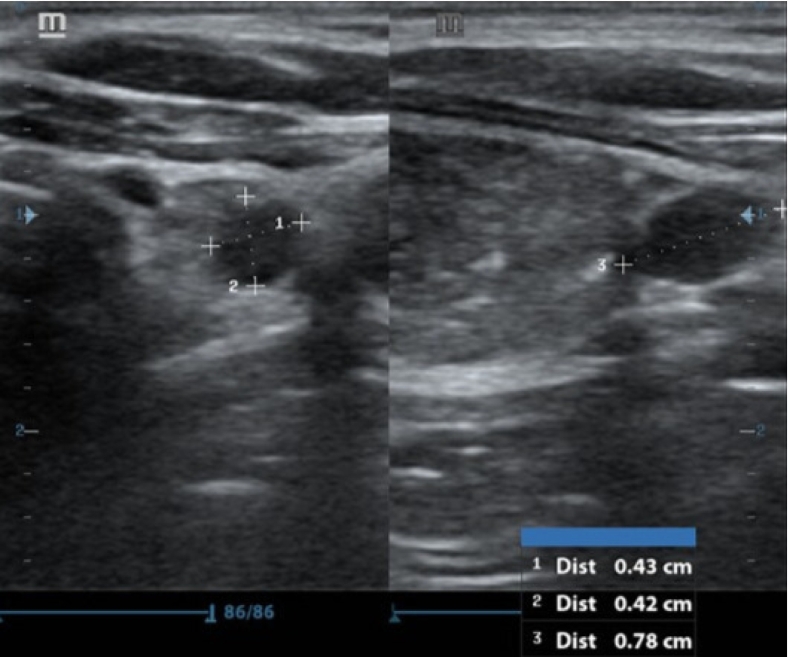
Ultrasound-guided exploration of the neck, showing a well-defined, homogenous, hypoechoic, oval-shaped solitary nodule below the lower pole of the left thyroid lobe, visualized from different angles.
